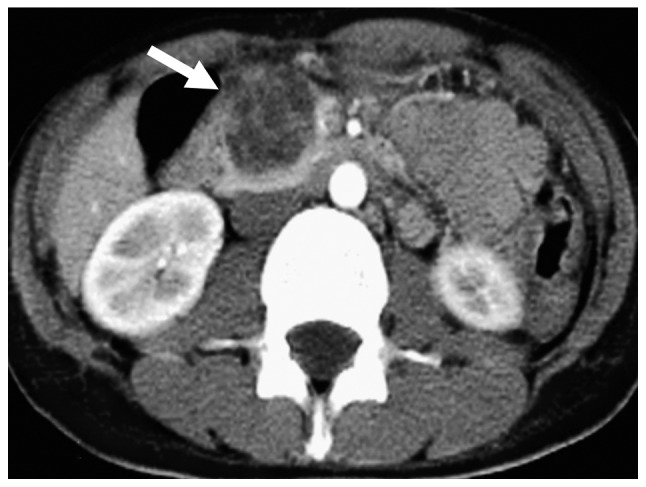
Enhanced abdominal computed tomography on admission demonstrating a tumor containing a cyst-like low-density area and an enhanced septum (arrow).
